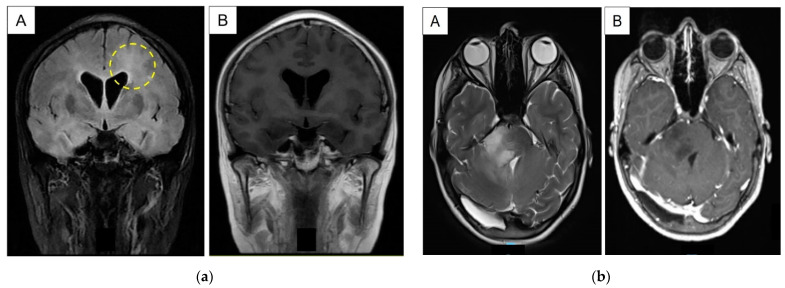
(a) Representative MRI coronal images in fluid attenuated inversion recovery (FLAIR) and post-contrast T1-weighted sequences, labelled (A) and (B) respectively. The decision was made for the biopsy to be taken adjacent to the left frontal horn (marked out by yellow circle in dashed lines) where there were more FLAIR-attenuated changes. (b) Representative MRI axial images in T2-weighted and post-contrast T1-weighted sequences, labelled (A) and (B) respectively. The patient underwent stereotactic needle biopsy of the right pontine lesion.
